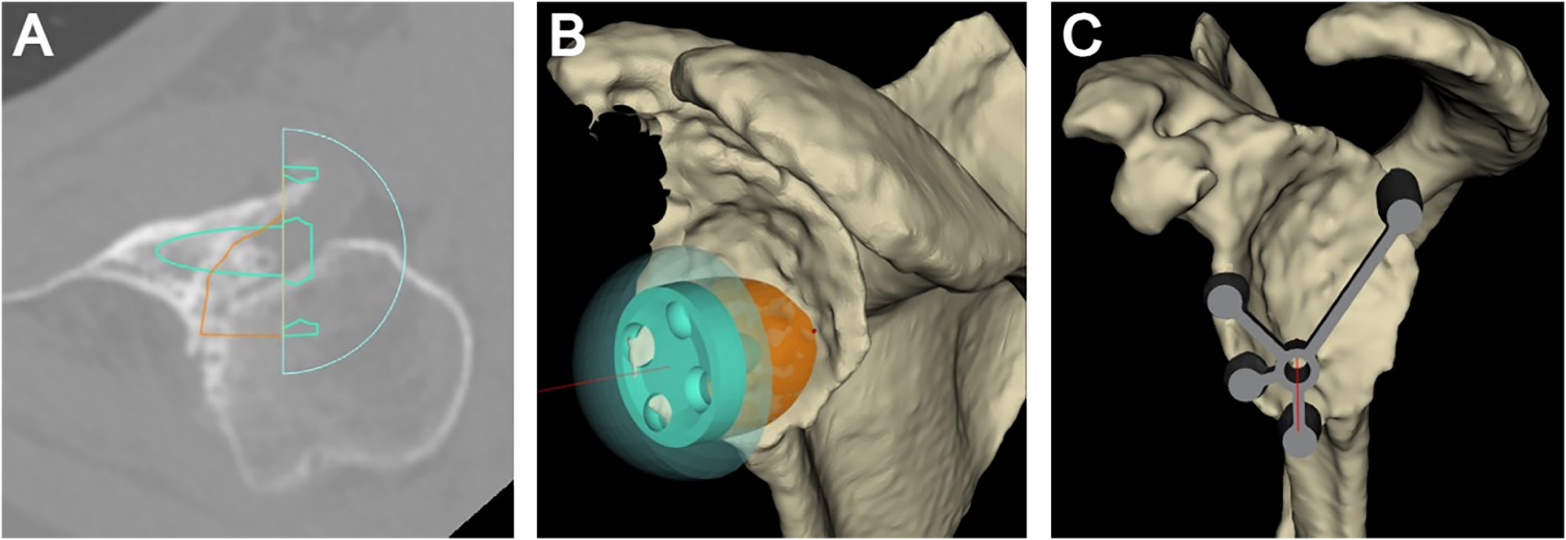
Case example (advanced CTA, left shoulder) outlining the necessary steps taken for preoperative planning. Three-dimensional (3D) planning software reveals 38° of glenoid retroversion and 5° of inferior inclination. (A) The planning software is used to determine the optimal position of the glenoid baseplate in the axillary (ie, the primary plane of deformity in this case) and coronal planes. (B) 3D rendering that includes the patient-specific bone graft (ie, digital graft) with the “point of maximal glenoid defect” denoted by the red dot. (C) The planned location and orientation of the glenoid guide pin are templated. Note is made of the substantial glenoid retroversion.
